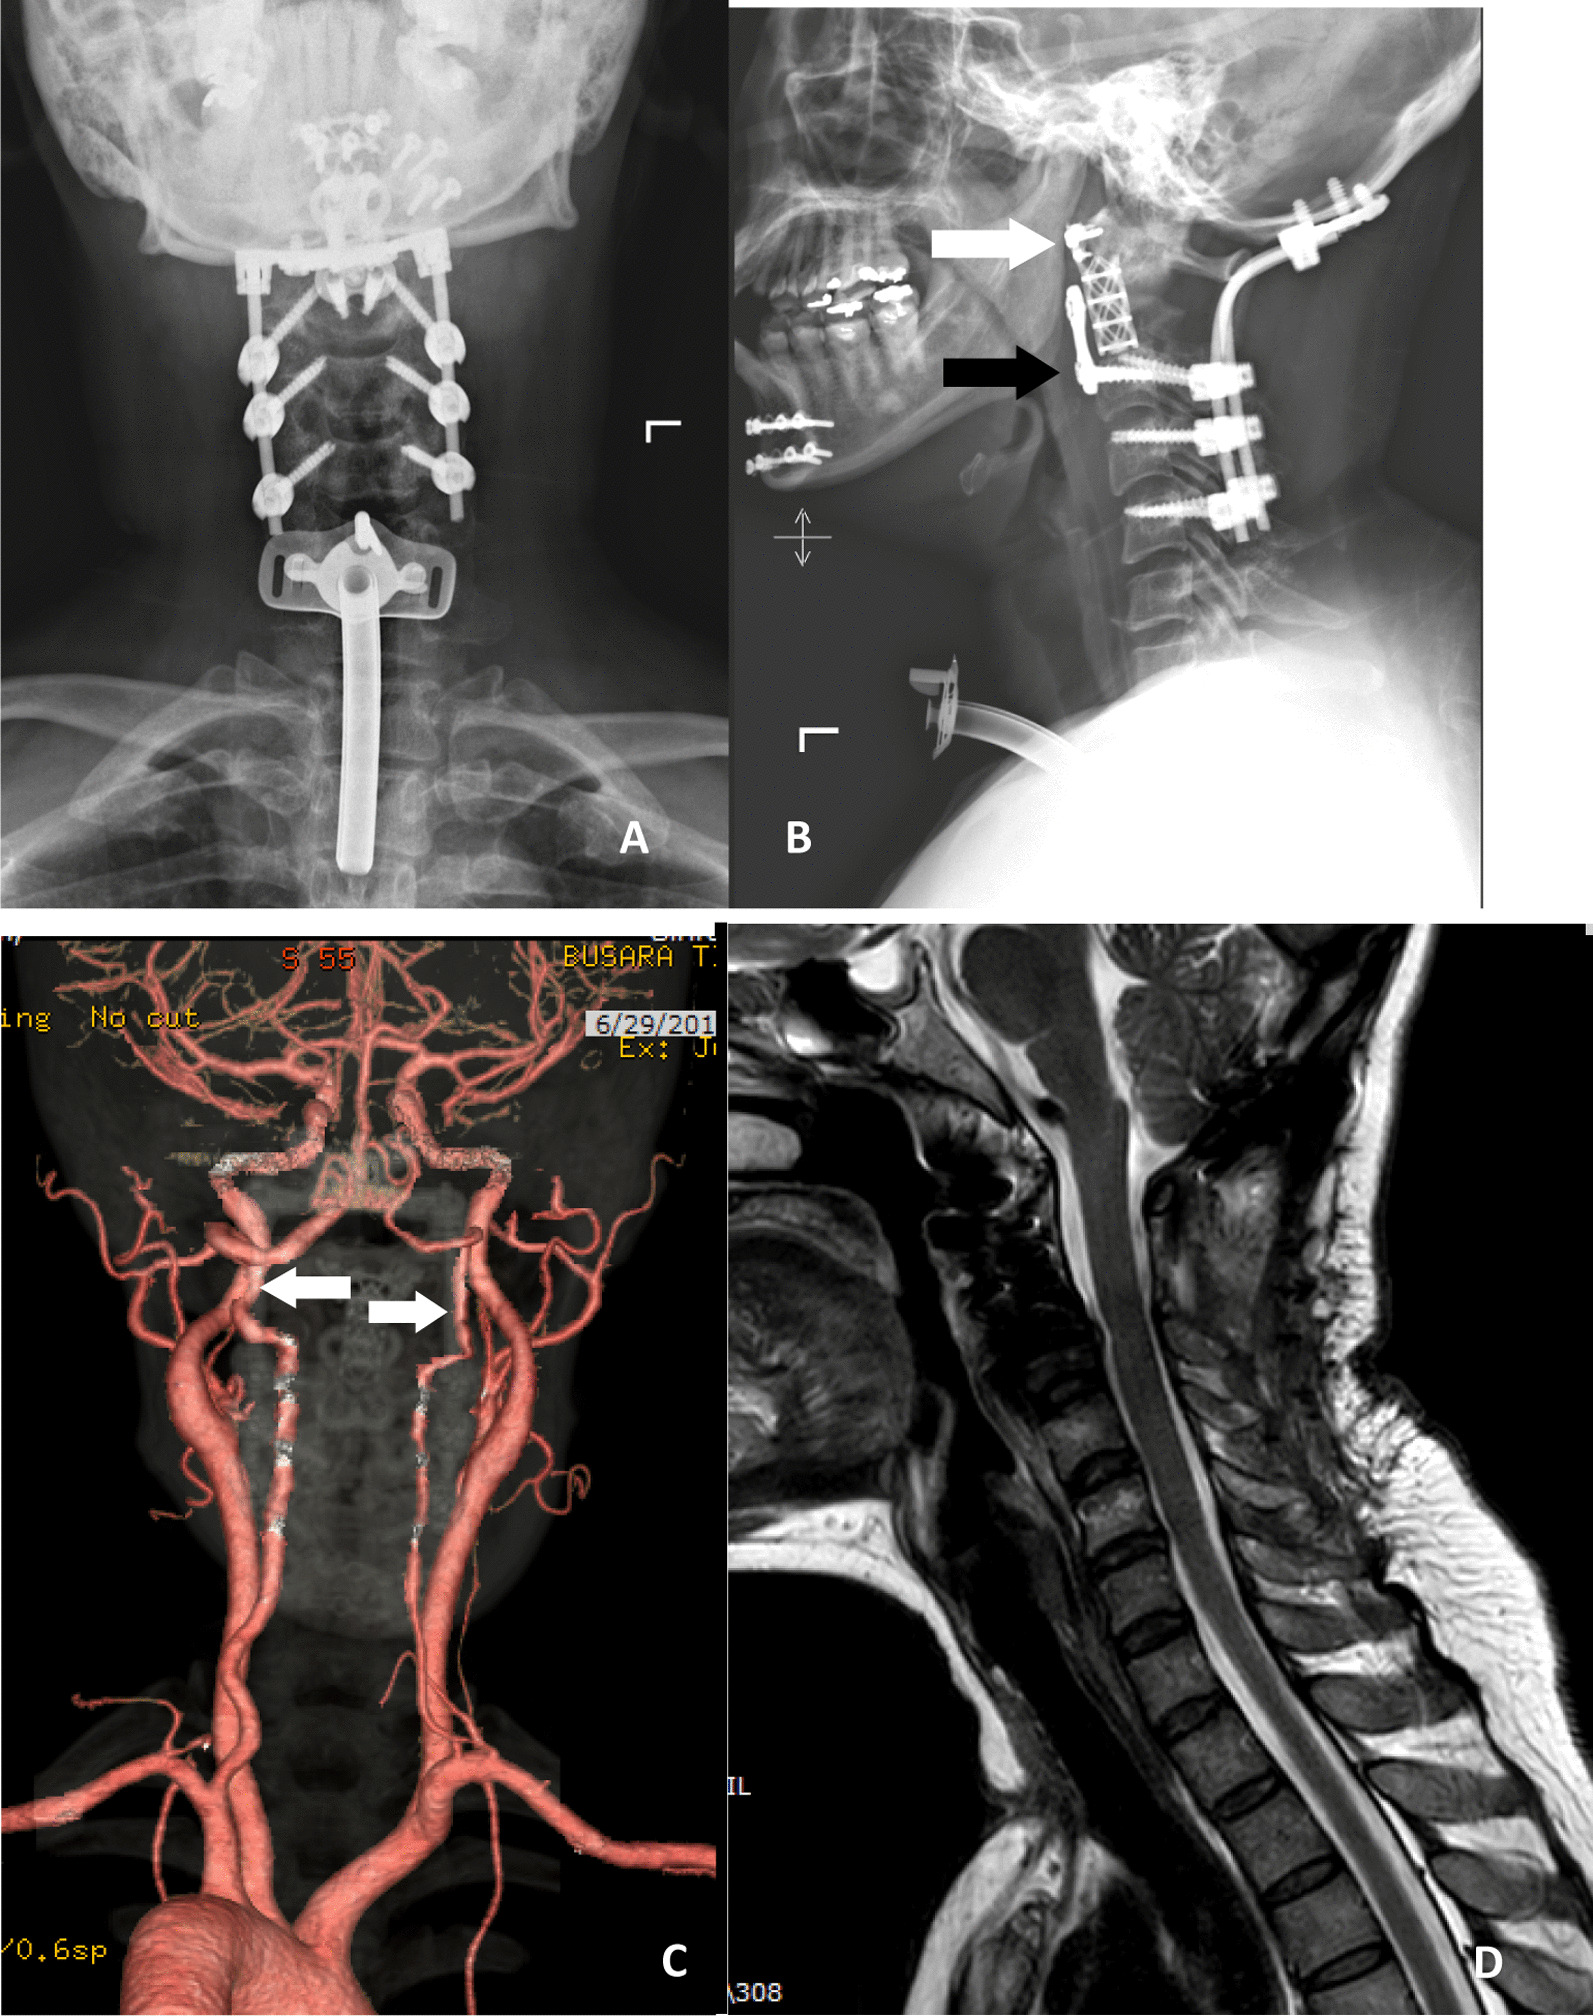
An immediate postoperative plain radiograph of the cervical spine showing the titanium mesh cage (white arrow) with the anterior kick-off plate (black arrow) and posterior instrumentation from the occiput to C5 (A, B). Patency of the bilateral vertebral arteries was apparent upon computed tomography angiography (white arrow) (C). A T2-weighted sagittal cervical spine MRI revealed no tumor mass and demonstrated an intact spinal cord (D)
